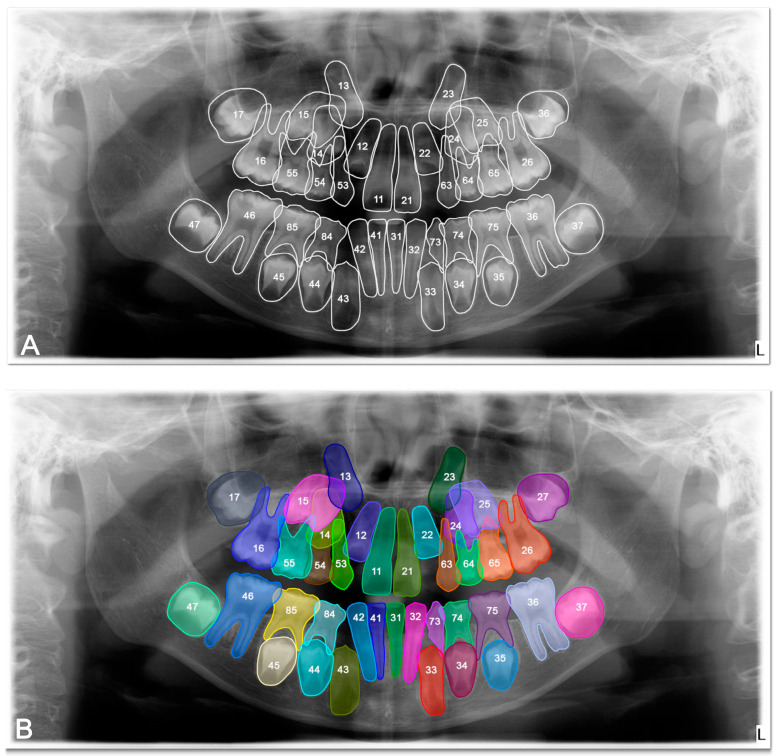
Images demonstrating the performance for tooth agenesis detection on panoramic radiographs between manual annotation by three dentists and U-Net based artificial intelligence (AI)-predicted label masks. (A) Manual segmentation performed by clinicians. (B) Automatic segmentation by the model. In the segmentation figures, each tooth is represented with a distinct colour to visually differentiate regions; however, the model treated all teeth as unified segmentation classes during training.
